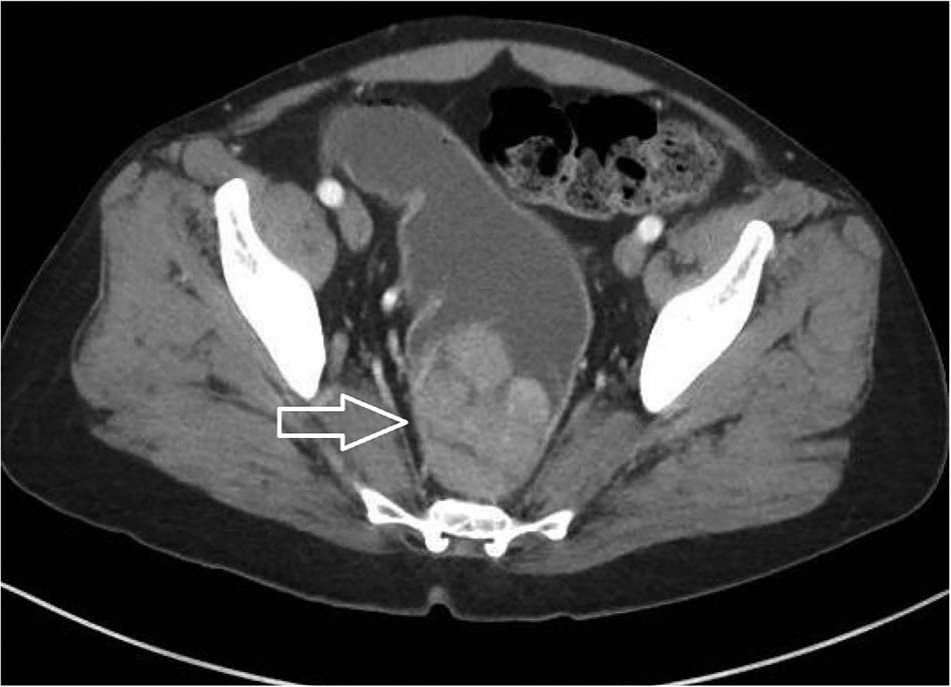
Axial section of the abdominal and pelvic CT showing large villous rectal mass measuring 9.6 cm (white arrow).
